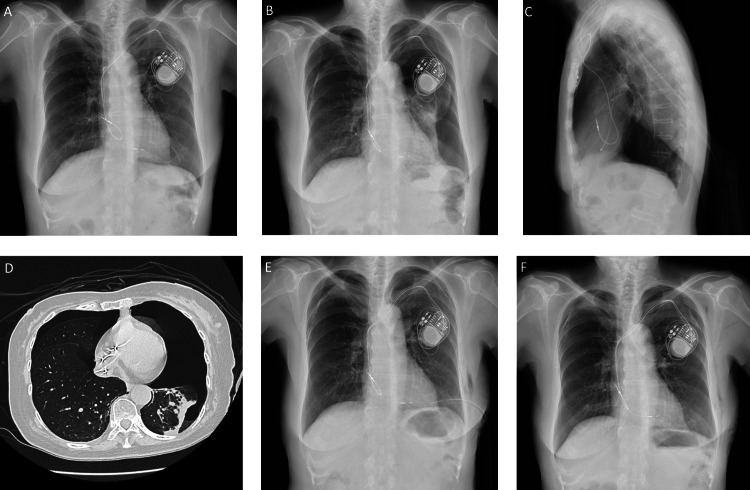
Serial changes in chest imaging Chest radiograph showed no pneumothorax 20 minutes after implantation (A), but a left-sided dominant bilateral pneumothorax is noted (B-D). A follow-up chest radiograph obtained three days after the insertion of the chest tube to the left chest shows improvement of pneumothorax in severity on both sides (E). The next day after the removal of the chest tube, only a mild pneumothorax is shown on the right side (F).
